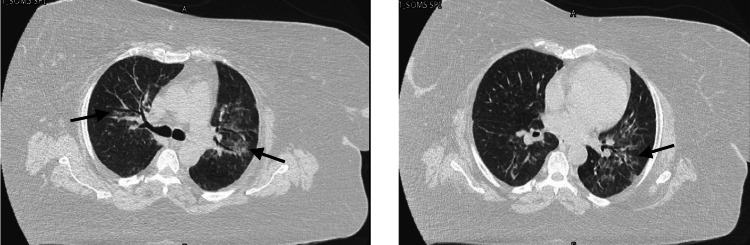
High-resolution CT of the chest demonstrates bilateral patchy ground-glass opacities predominantly involving the upper lobes (left panel), with more pronounced subpleural ground-glass opacification in the left lower lobe (right panel), as indicated by the arrows
